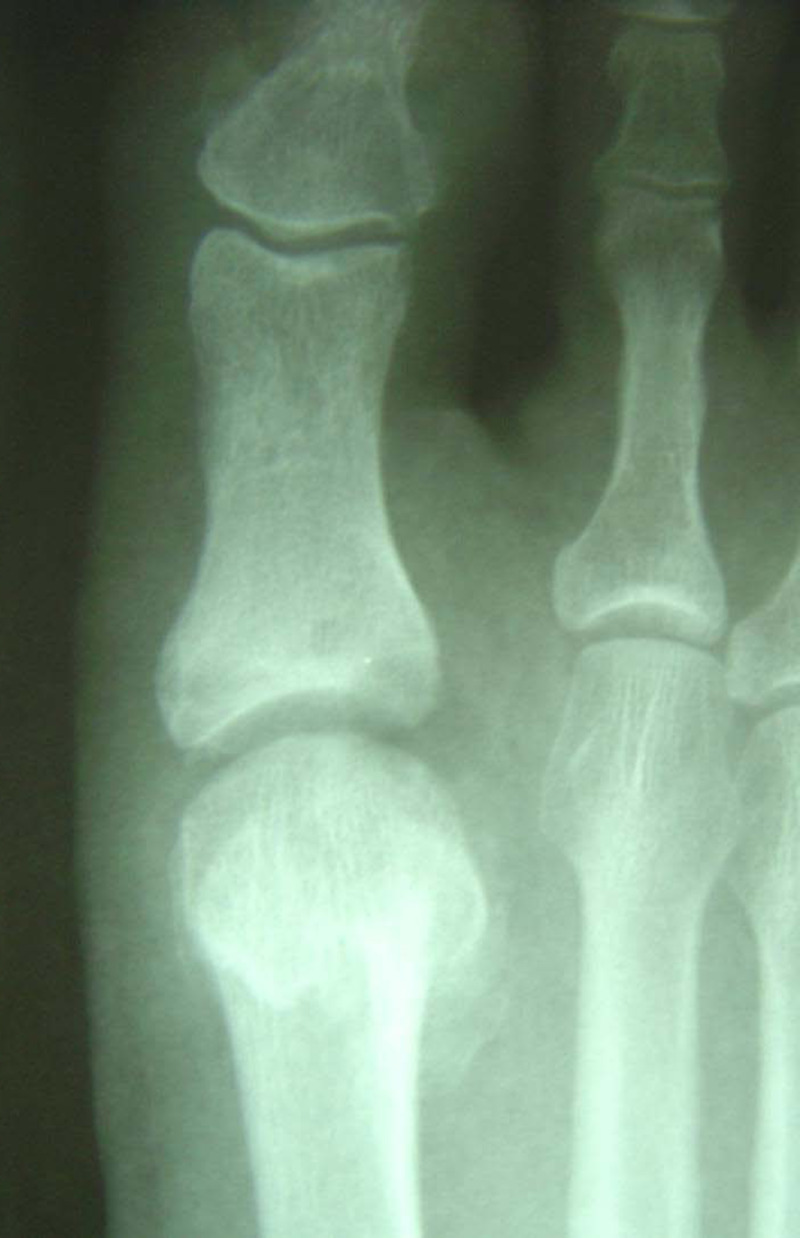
Six weeks postoperative radiographs demonstrating joint decompression
